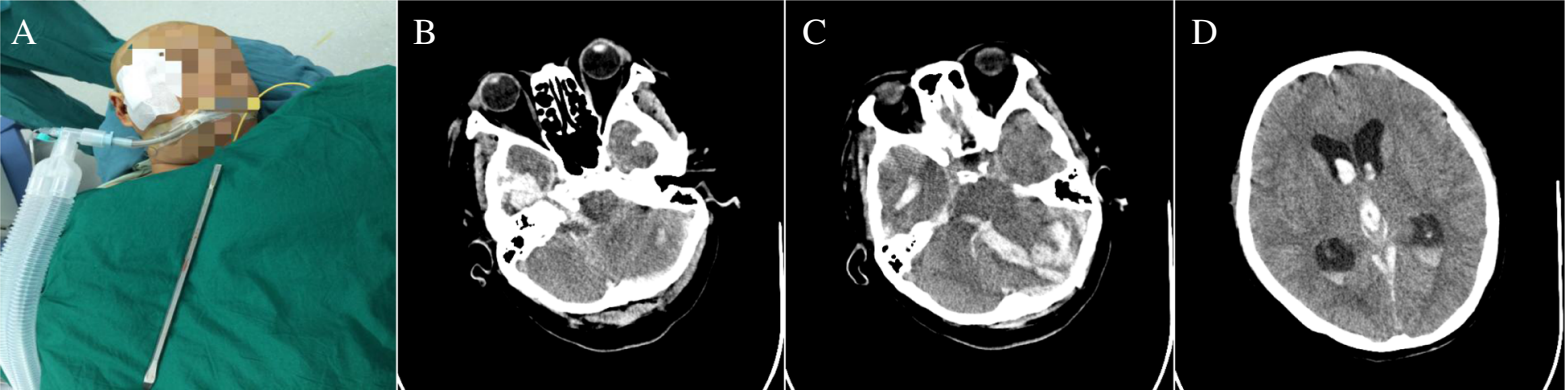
a. Clinical picture showing the strewdriver removed surgically. b. CT of head-brain window showing hematoma of strewdriver tract. c. CT scan showing a left cerebellar contusion with hematoma and obvious compression of the fourth ventricle and brain stem. d. CT scan showing high density corrosion cast of the ventricular system
